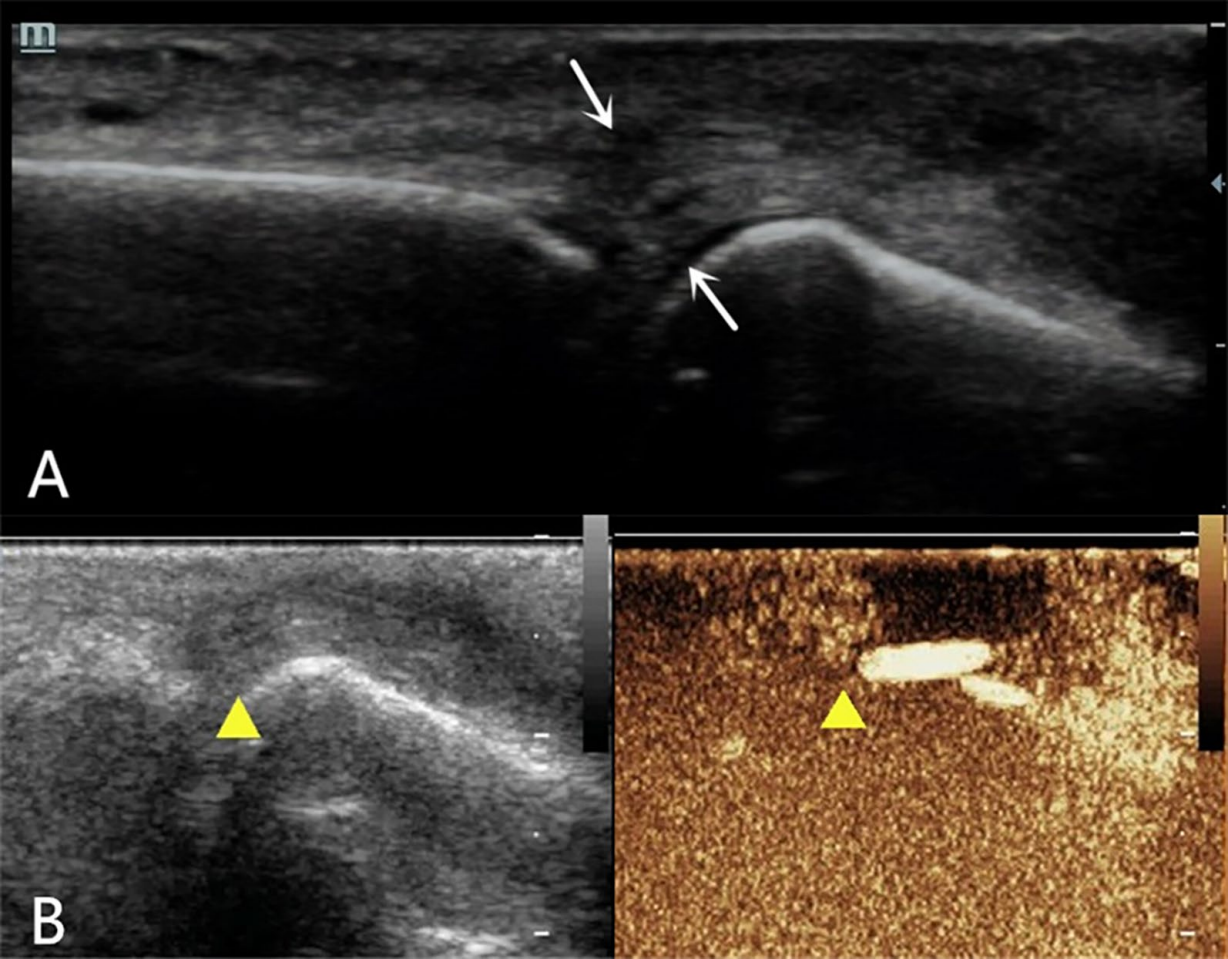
Ultrasound examination of a 52-year-old male with right fourth finger extensor tendon injury. A Two-dimensional ultrasound showed that the horizontal extensor tendon of metacarpophalangeal joint was thickened, with reduced echogenicity and a visible crease (indicated by white arrow) B. CEUS shows an “up and down through”  type filling perfusion of contrast agent inside the tendon (indicated by a solid yellow triangle)
